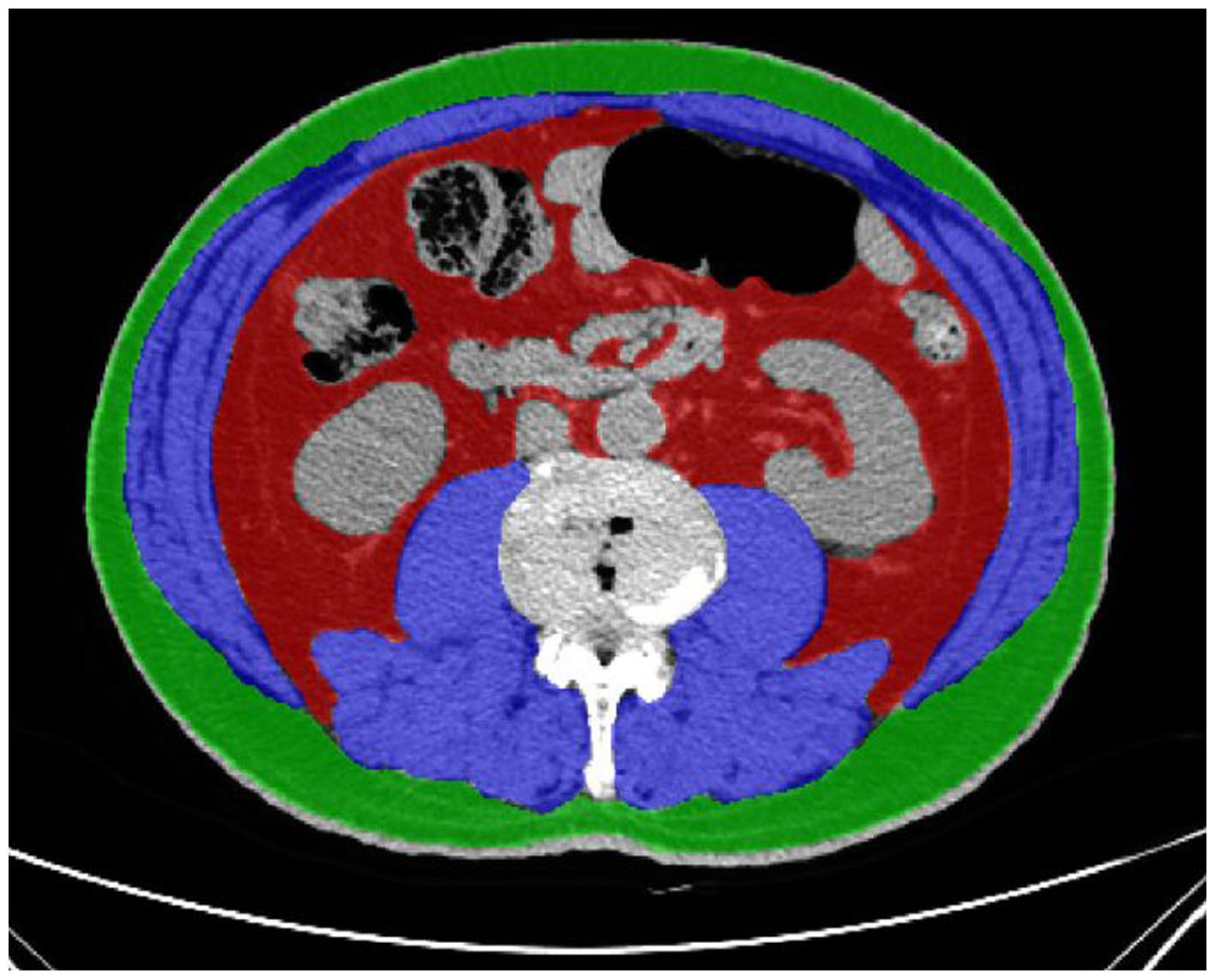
Cross sectional CT imaging at L3-L4 intervertebral disk. Muscle tissue is shown in blue color, subcutaneous fat tissue in green color, and visceral fat tissue in red color. Visceral fat tissue and subcutaneous fat tissue were identified and quantified using Hounsfield units (HU) thresholds of −150 to −50 HU and − 190 to −30 HU. Skeletal muscle was identified and quantified by use of HU thresholds (−29 to +150).
